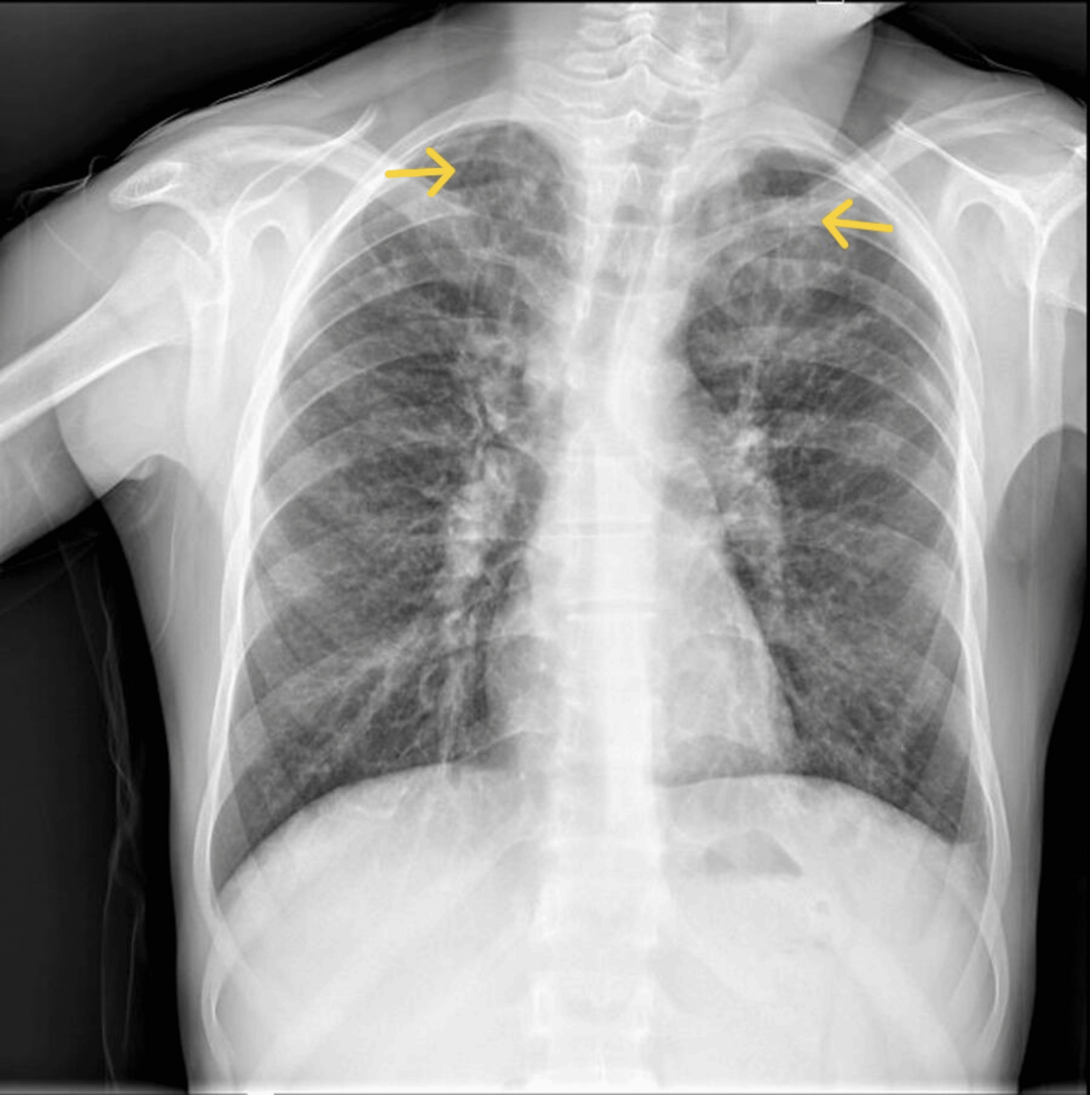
Frontal chest radiograph showing bilateral heterogeneous infiltrates and multiple cavitary lesions, most pronounced in the upper lobes. Arrows indicate the cavitations.
